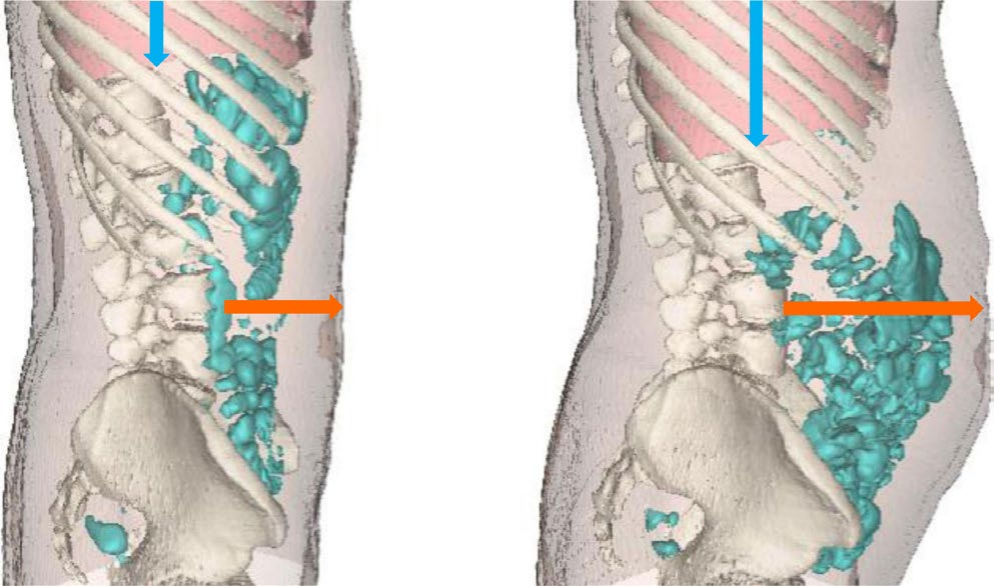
Abdominal CT image in a patient during basal conditions and during an episode of lettuce‐induced distension. Note that abdominal distension is associated to a diaphragmatic descent (blue arrow) and anterior wall protrusion (orange arrow) without substantial increase of intestinal gas
